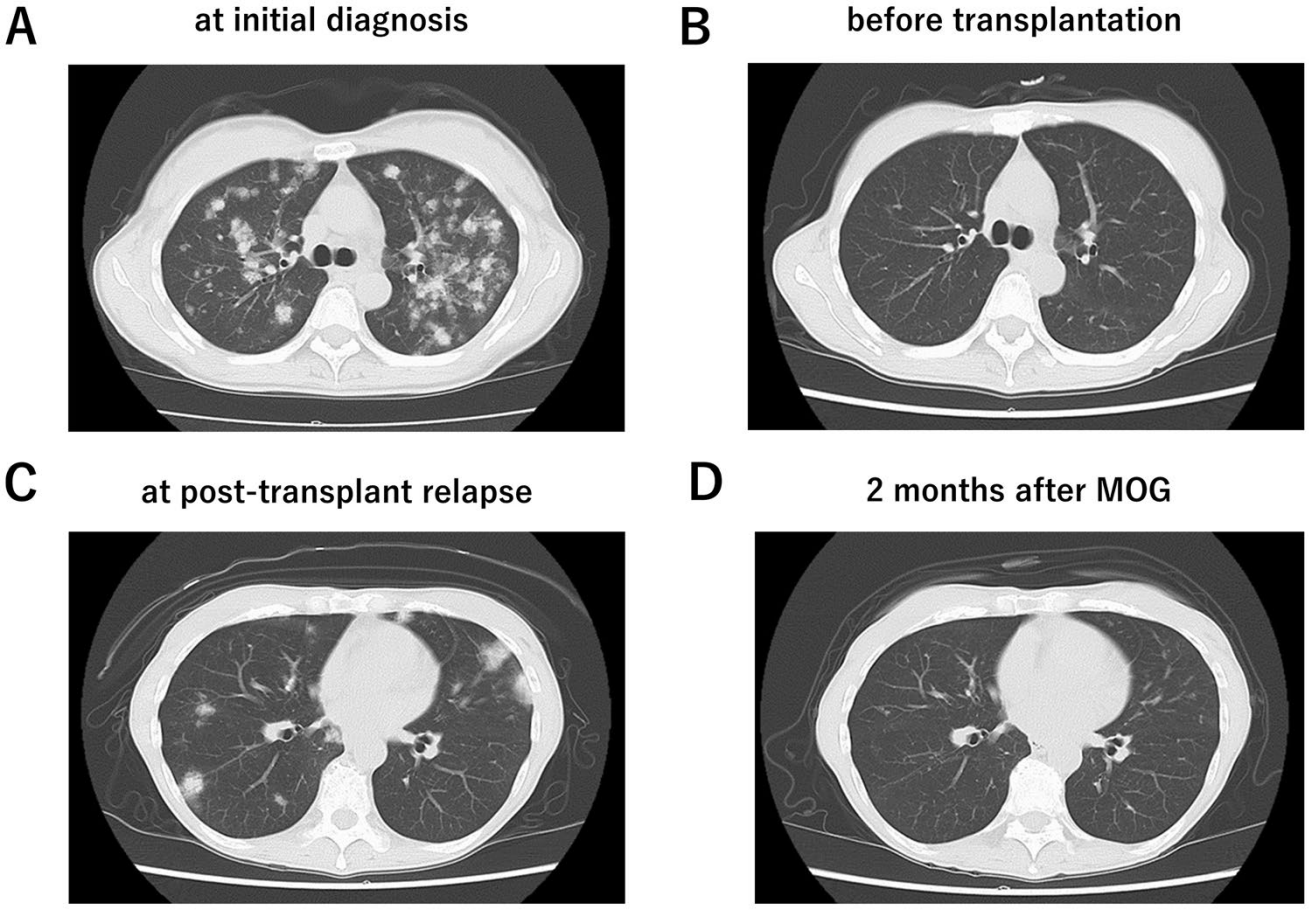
Computed tomography (CT) images of the chest at the initial diagnosis of adult T-cell leukemia/lymphoma (ATLL) (A), before bone marrow transplantation (B), during post-transplant relapse (C), and 2 months after mogamulizumab (MOG) therapy (D)
